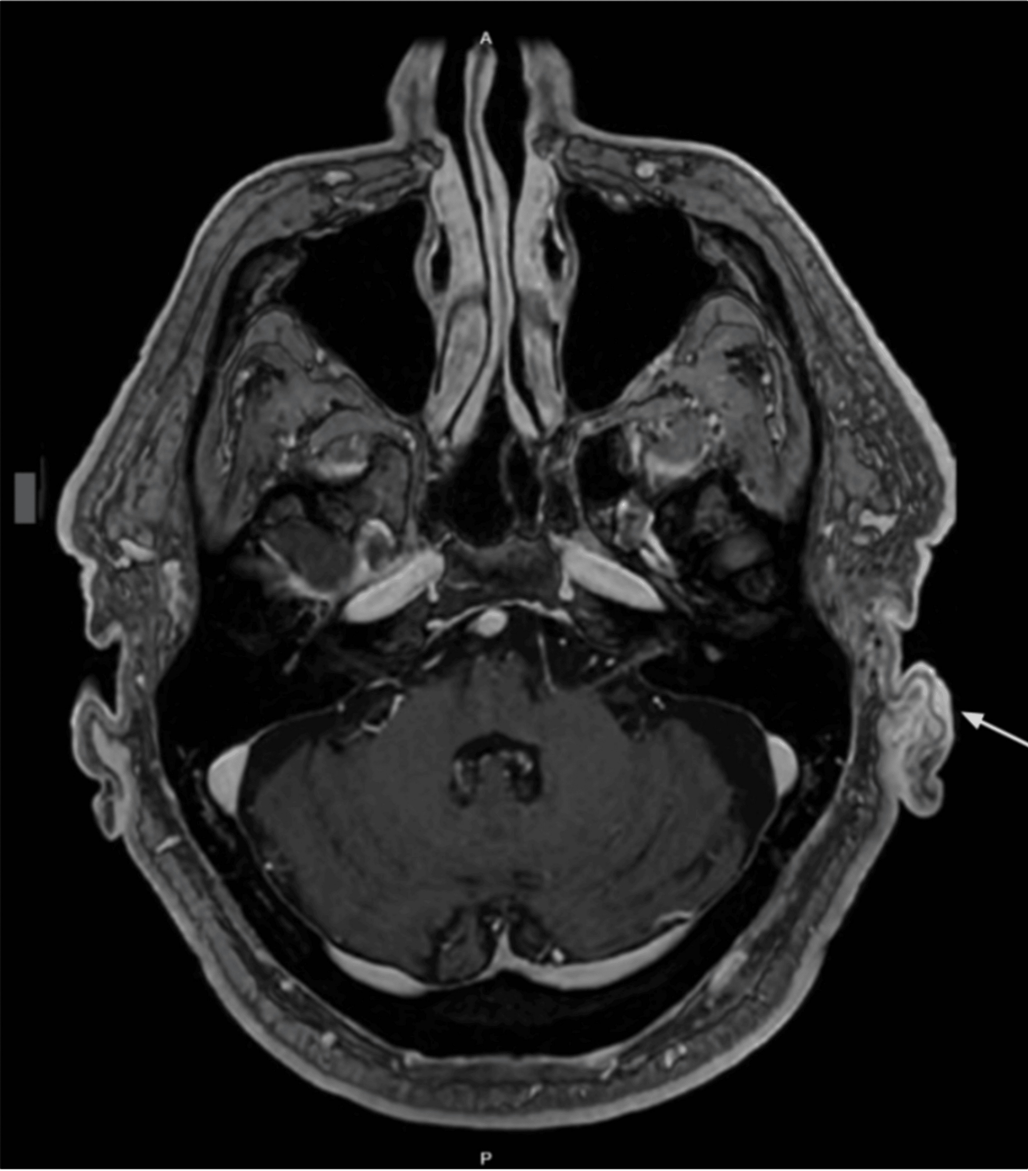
Axial post-contrast MRI demonstrating enhancement of the left external ear and periarticular soft tissues, consistent with active varicella-zoster virus (VZV) reactivation.
